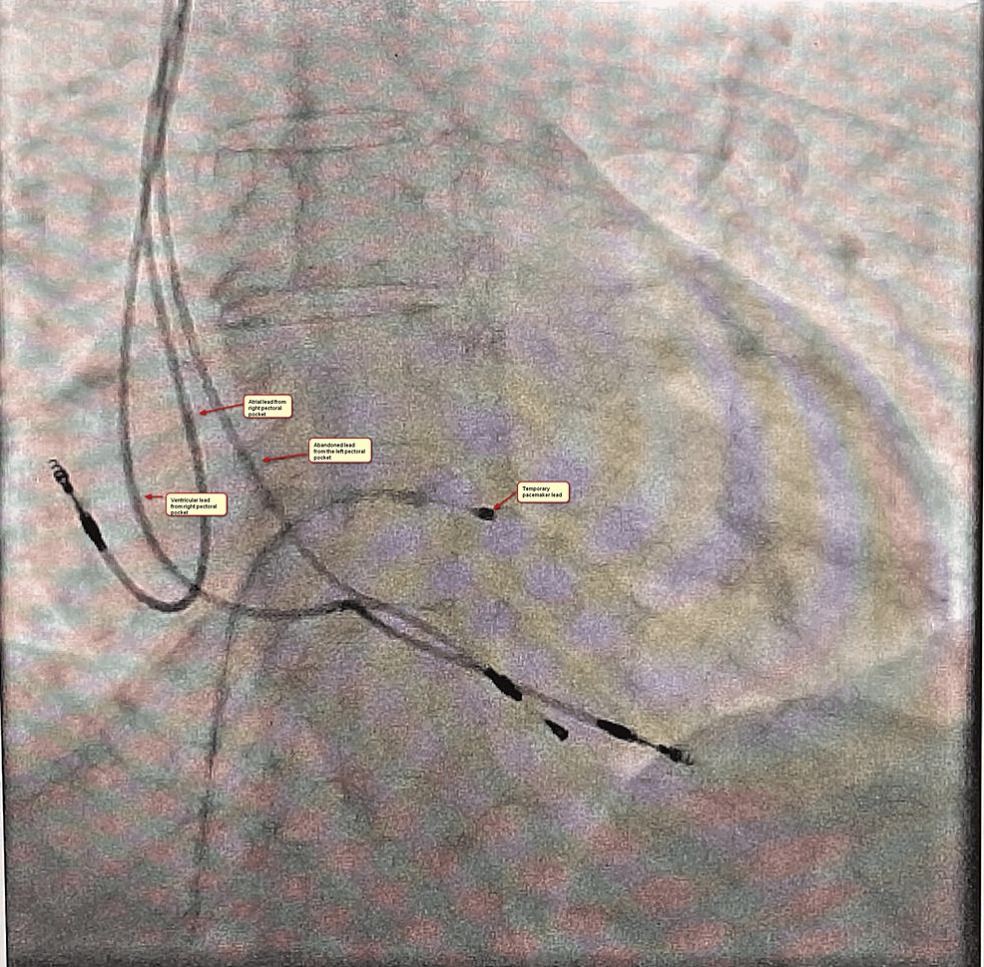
Chest x-ray Chest x-ray (posterior-anterior (PA) view) shows an abandoned left-side lead and two leads from the right-sided infected pacemaker pocket.
